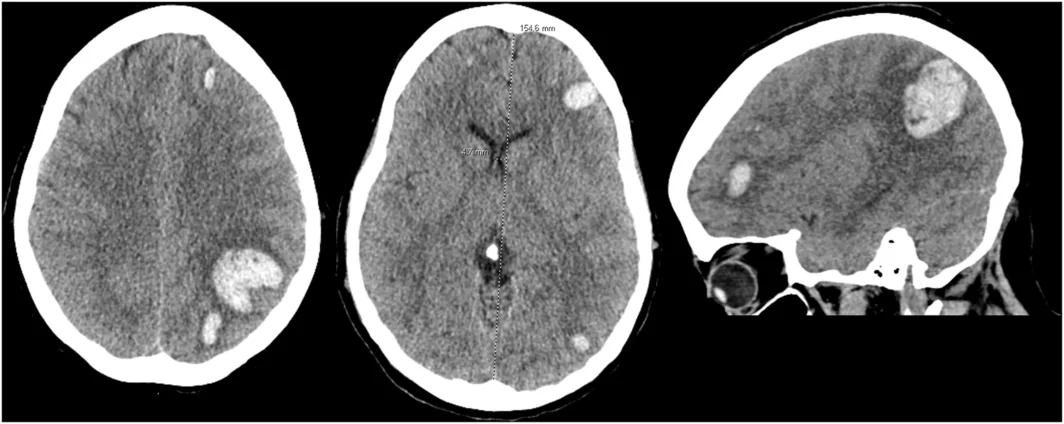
Pretransplant brain CT demonstrating multifocal intraparenchymal hemorrhages with rightward midline shift.
